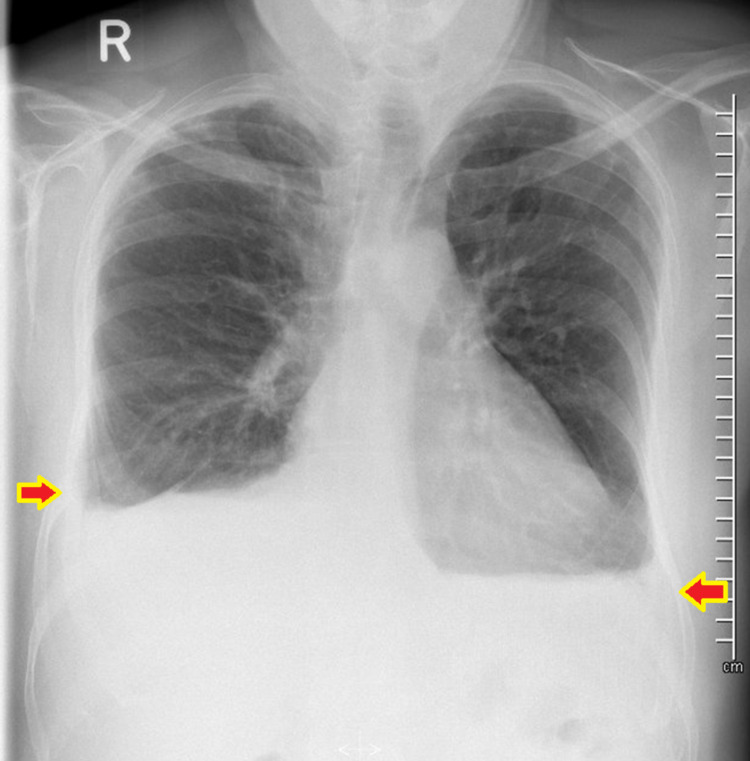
Chest x-ray showing bilateral pleural effusion and blunting of both costophrenic angles (August 2020)
